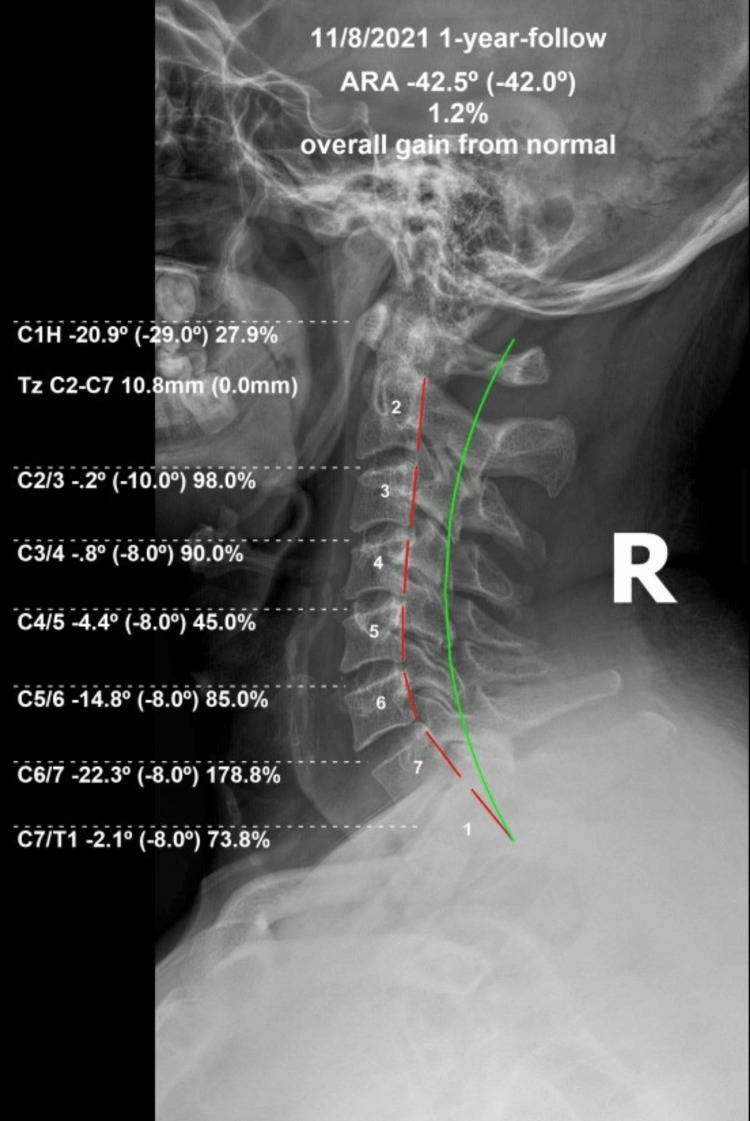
Long-term lateral cervical radiograph with stability The dashed red line is the posterior body tangents showing the lordosis maintained at follow-up.  The green line represents the ideal cervical lordosis.
